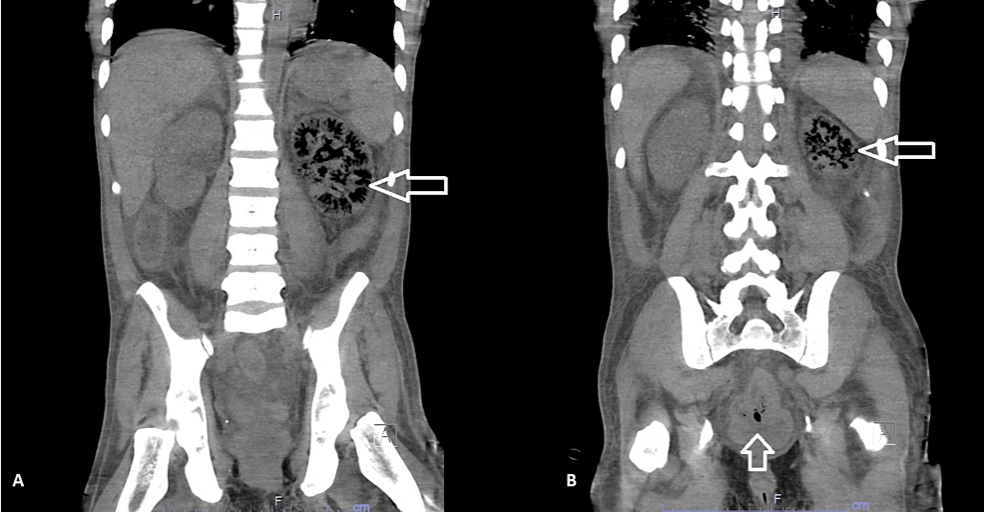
First CT Scan of the Abdomen and Pelvis Showing Extensive Gas Within the Left Renal Collecting System and Parenchyma Along With Intravesical Gas
